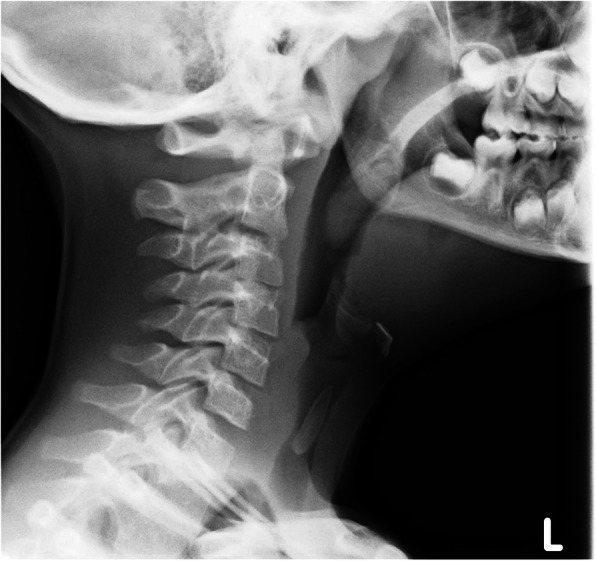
Lateral neck x-ray demonstrating foreign body lodged in upper trachea
